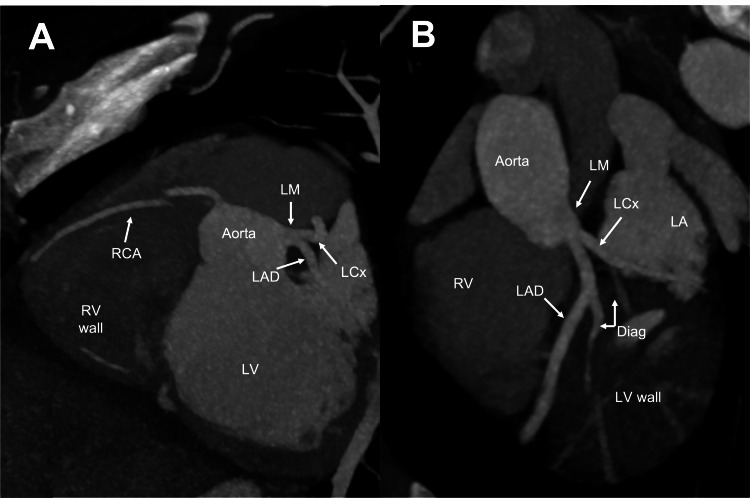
Coronary computed tomography angiography during hospitalization A short left main (LM) artery and right coronary artery (RCA), cut-off due to motion artifact in A, are seen branching off the aorta. The left main (LM) artery bifurcates into the left circumflex artery (LCx) and the left anterior descending artery (LAD). The diagonal branches (Diag) are seen coming off the left circumflex artery (LCx) and the left anterior descending artery (LAD) in B. The coronaries are normal with a zero calcium score. LA: left atrium, RV: right ventricle, LV: left ventricle.
